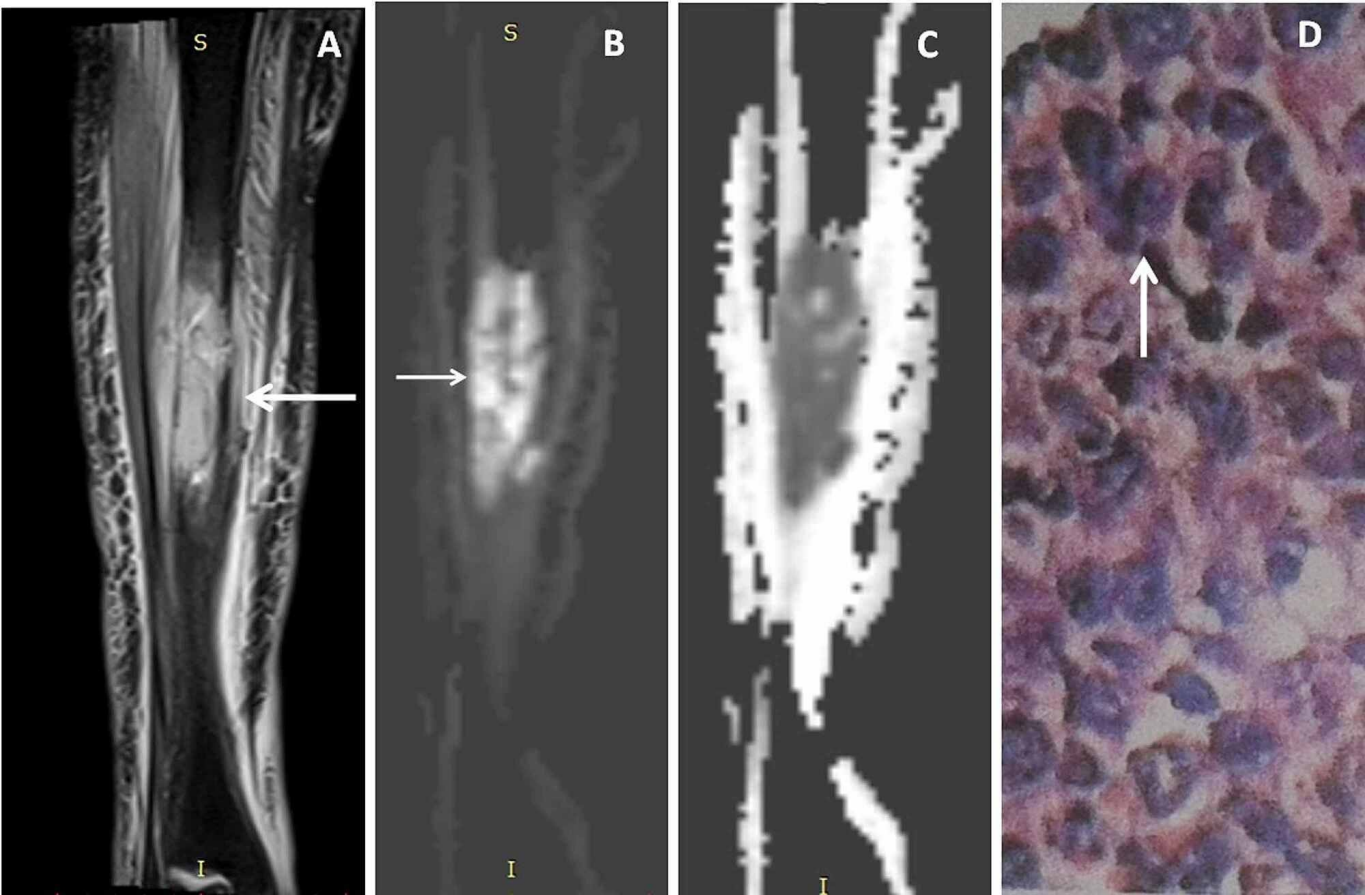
Tibial shaft lymphoma Forty-seven-year-old female with right leg swelling. Coronal proton density fat-suppressed (PDFS) image (A) showed minimally expansile intramedullary lesion in middle 3rd of tibial shaft with destructions of bony cortices and surrounding soft tissue infiltrations (←arrow). Coronal diffusion-weighted image (DWI) (B) and apparent diffusion coefficient (ADC) map image (C) showed diffusion restriction (→ arrow) with low ADC value. 40X HPE image (D) showed bony tissue infiltrated by tumor composed of small round to oval cells, (↑ arrow) where the cells showed vesicular nuclei with prominent nucleoli. Immunehistochemistry confirmed diagnosis of non-Hodgkin's lymphoma with positive leucocyte common antigen (LCA) and negative cytokeratin and desmine.
